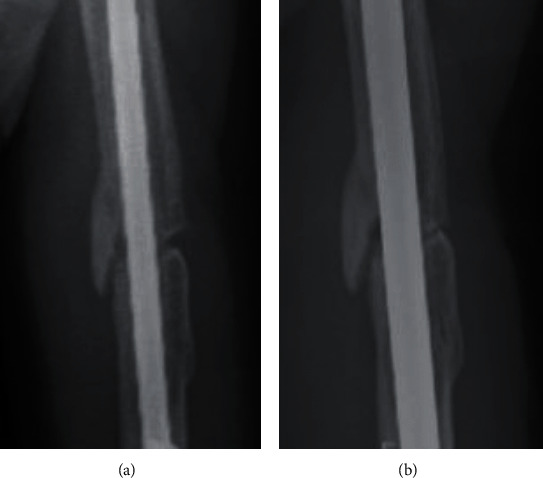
Radiographs comparing the gap before and after the nonunion surgery. (a) The gap before the surgery and (b) the gap after the surgery. It can be noted that the gap was reduced by compression.
